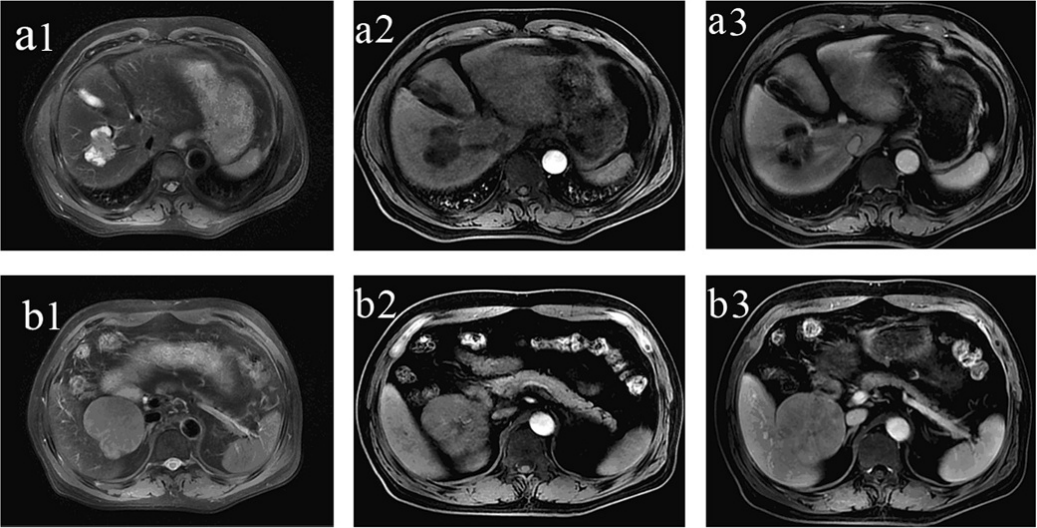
Abdominal magnetic resonance imaging depicted two isolated tumors. One measured 4.0 cm in diameter and was present in the left segment of the liver (SVIII, a1). The other measured 6 cm in diameter and was present in the inferior superior segment of the liver (SV, b1). The larger tumor showed enhancement (b2), and the smaller tumor showed contrast enhancement (a2) in the center of the tumor in the hepatic arterial phase. In the portal phase, the larger tumor became less dense than the liver parenchyma (b3), but the centrality of the smaller tumor showed continuous enhancement (a3).
